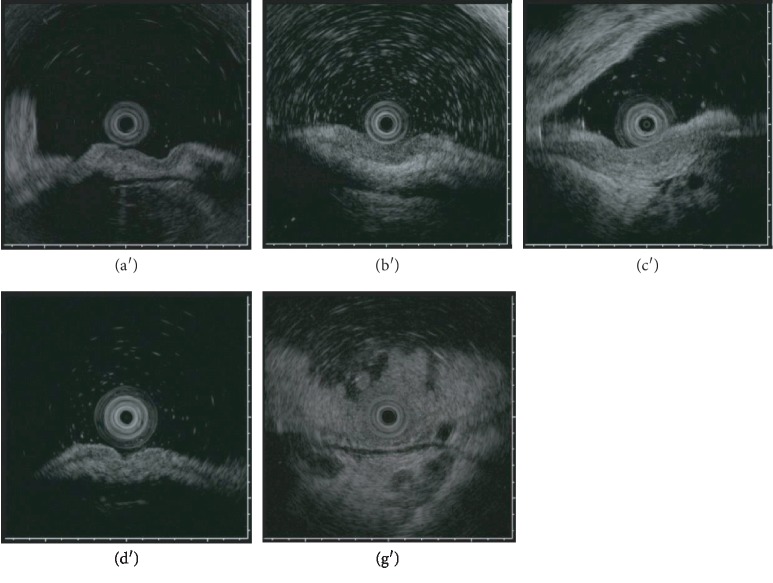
Endoscopic ultrasonography images of 5 lesions: (a′) lesion ① in Tables 1–4; (b′) lesion ② in Tables 1–4; (c′) lesion ③ in Tables 1–4; (d′) lesion ④ in Tables 1–4; (g′) lesion ⑦ in Tables 1–4.
